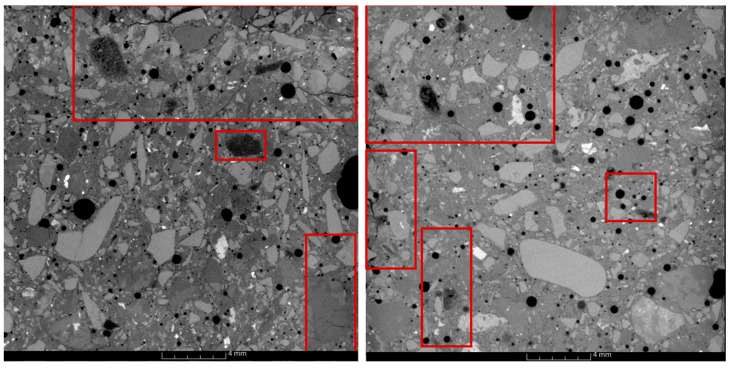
Example cross-sections of mortars with reactive aggregate obtained by computed tomography with red-framed cracks and corrosion centers.
